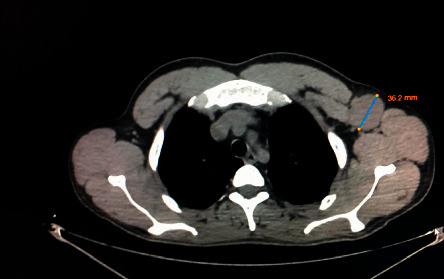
CT scan postoperatively showing a soft tissue lesion measuring 36.2 mm in the left axilla consistent of lymph node involvement.
